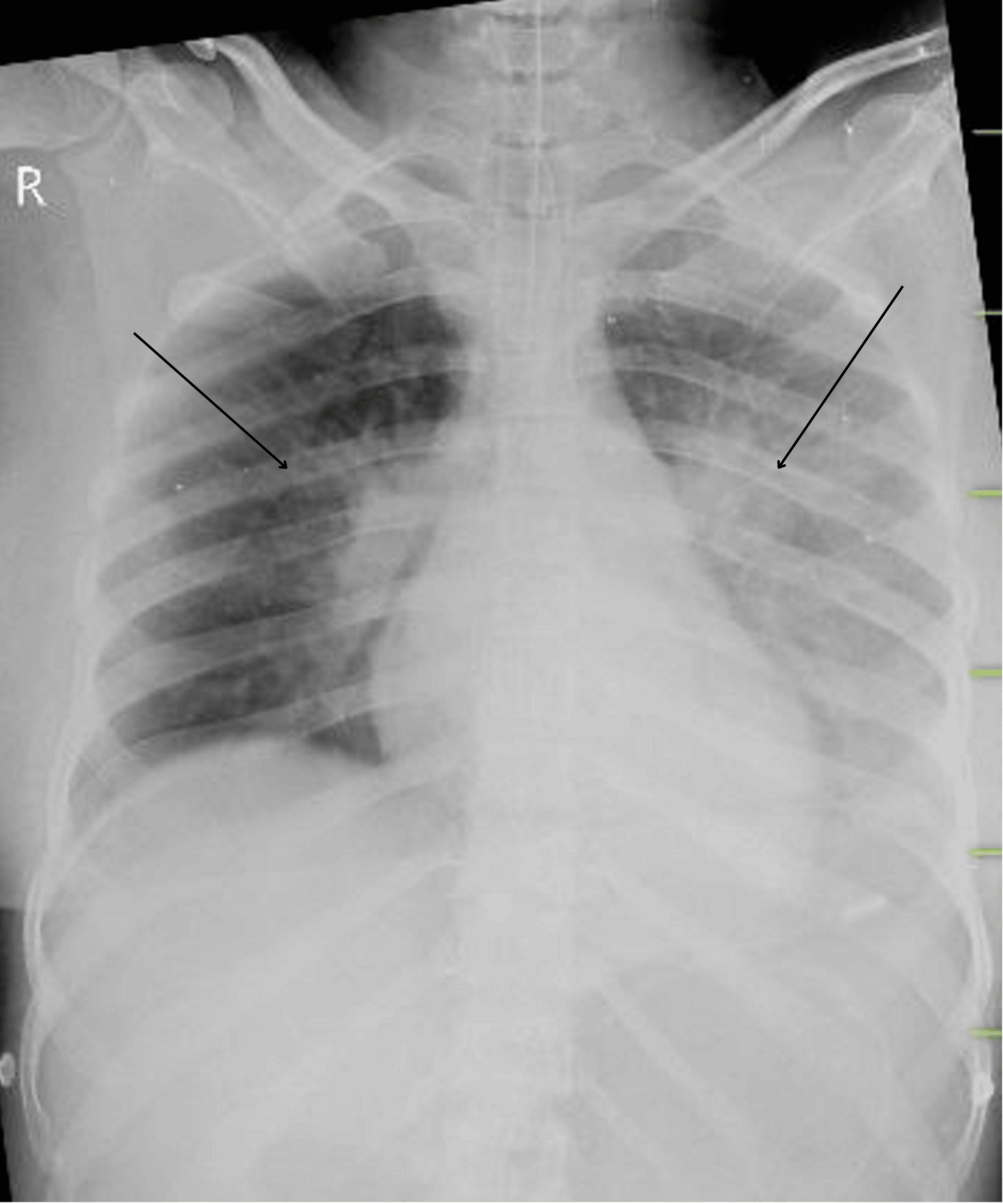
Chest radiograph of the patient showing hydrostatic pulmonary edema on the day of admission (black arrows)
